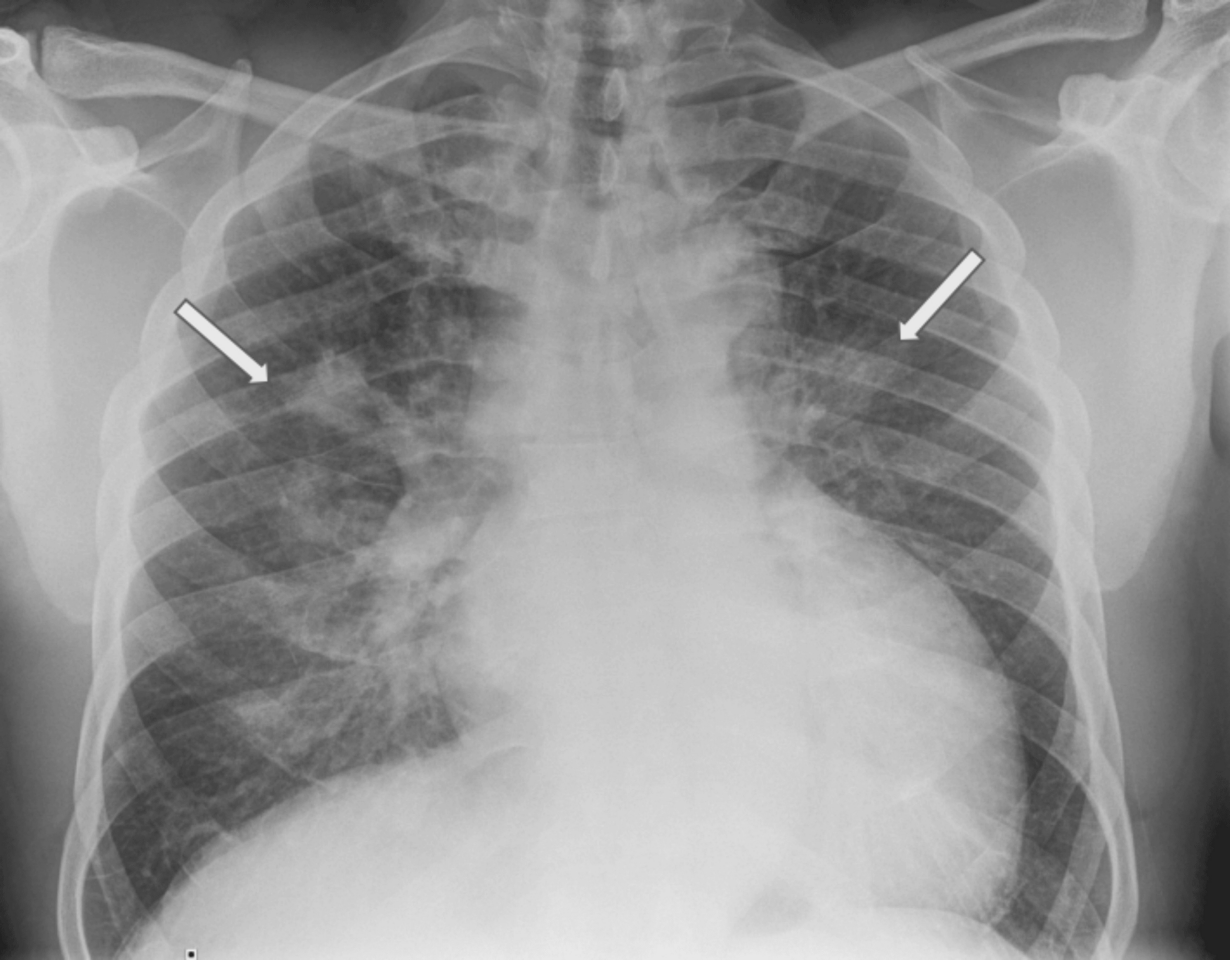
PA chest radiograph showing perihilar focal airspace shadowing (arrows) and increased cardiothoracic ratio (cardiomegaly).
